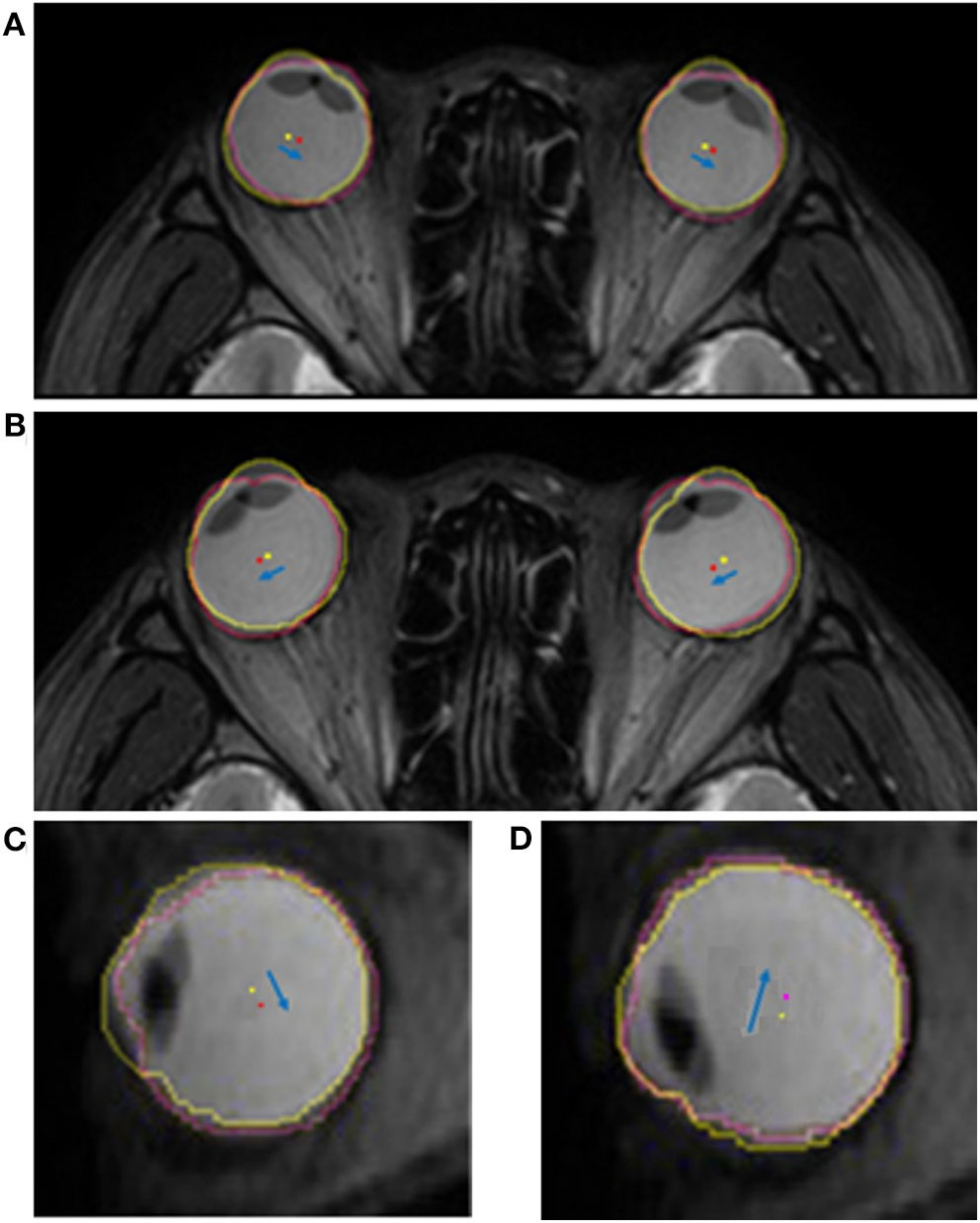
Superimposed MR images showing the translatory movements of the eyeball. The yellow and red dots indicate the centroid of the eyeball in the primary and secondary positions, respectively. The blue arrow indicates the movement of the centroid of each eyeball. Eyeballs move toward the gaze direction in the axial plane during horizontal gaze. (A) When the subject looks to the right, the centroids of both eyeballs translate to the right. (B) Similarly, when the subject looks to the left, the centroids of both eyeballs translate to the left. The translatory movement of the eyeball in the sagittal plane is in the opposite direction to the gaze during vertical gaze. (C) The eyeball is translated downwards when looking upwards, and (D) translated upwards when looking downwards.
